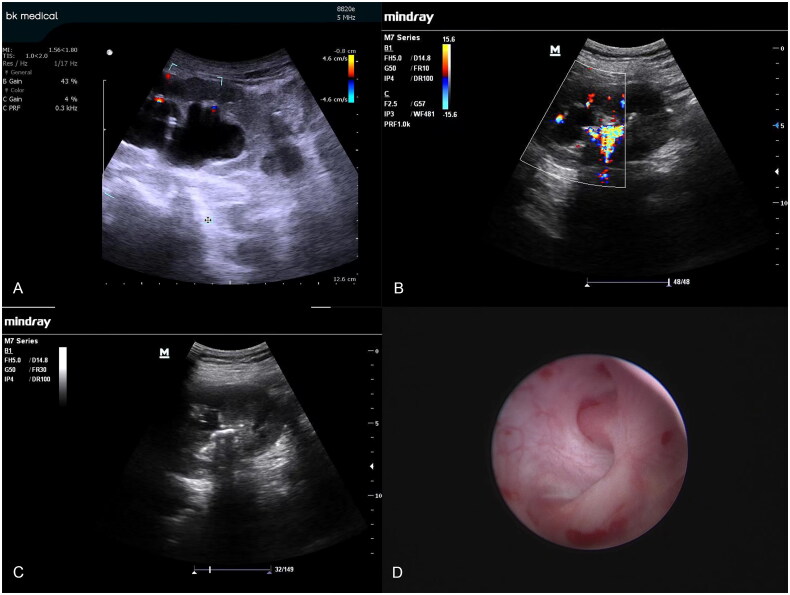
Color Doppler ultrasonography images of the fused calyceal kidney during percutaneous renal puncture (A,B), and the B-ultrasound image of the same fusion calyceal kidney (C). endoscopic morphology of the fusion calyces (D). Red indicates the artery; blue indicates veins.
